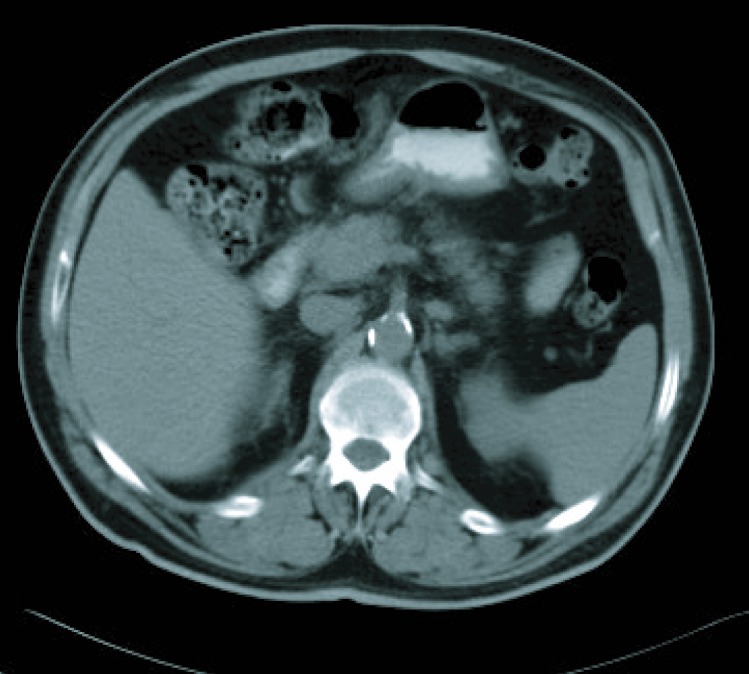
CT imaging after 6 cicles.
